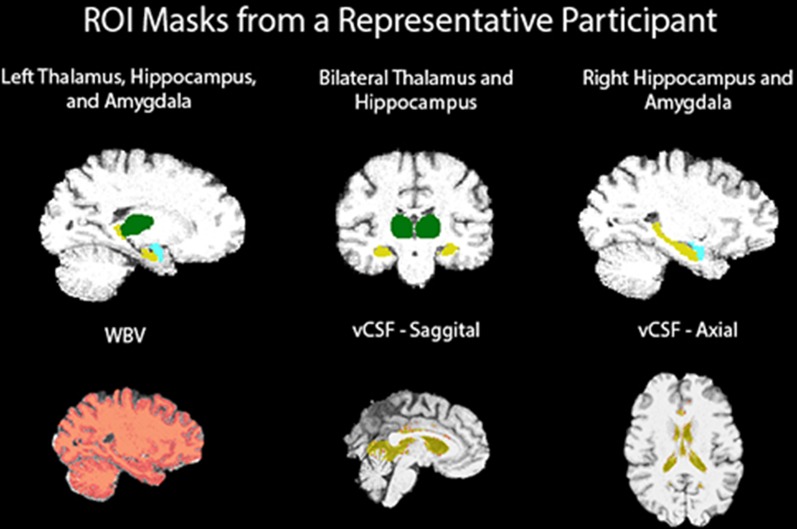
T1-weighted MRI ROI FIRST masks.
